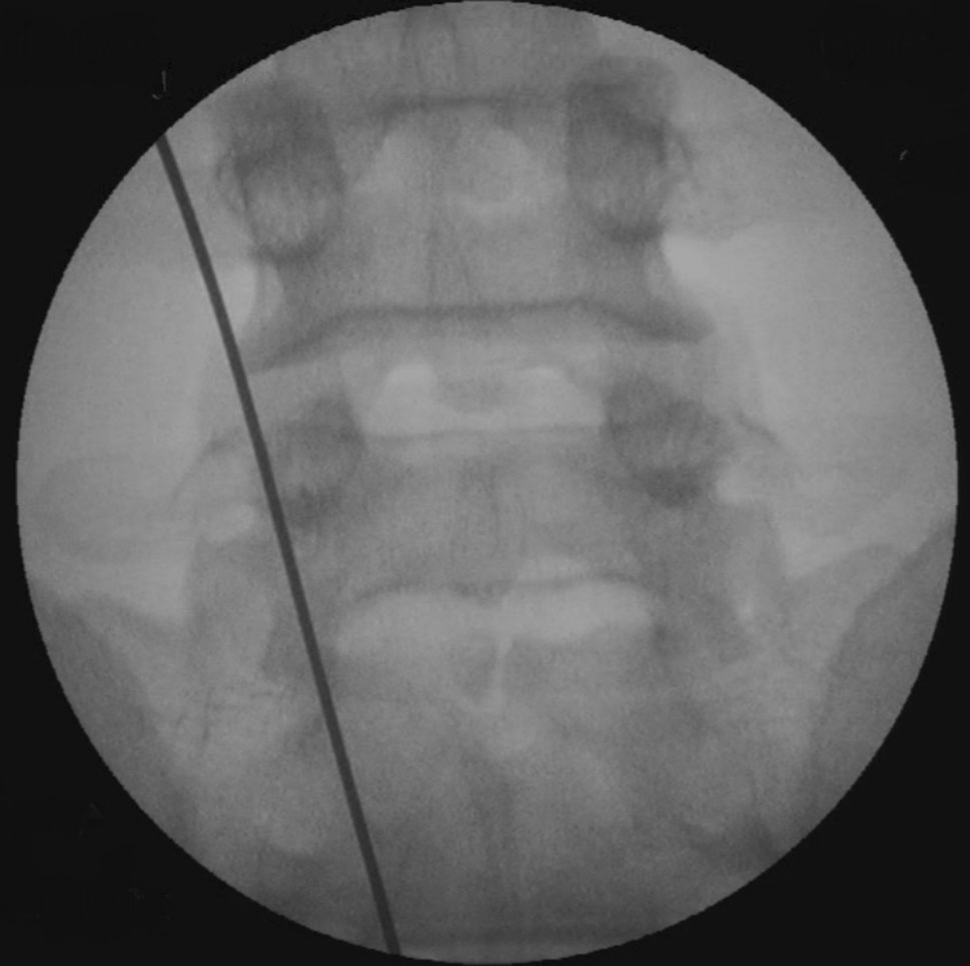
Wire placed over the pars interarticularis in AP view X-ray
